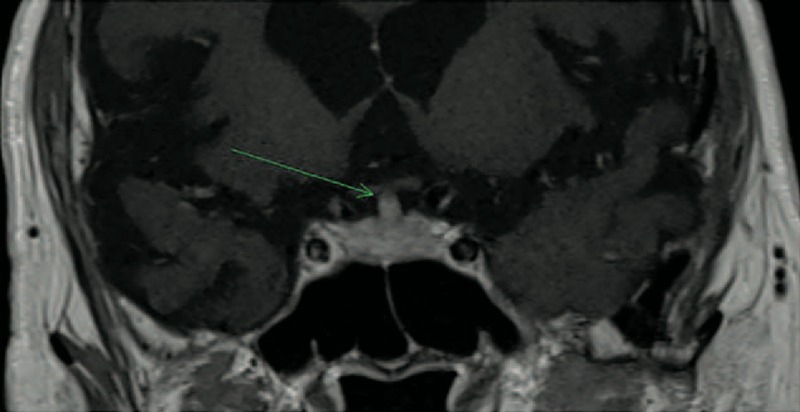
Patient 5 in 2014: coronal brain T1-weighted MR image after intravenous administration of gadolinium showing an enlargement of pituitary stalk (4 mm).
